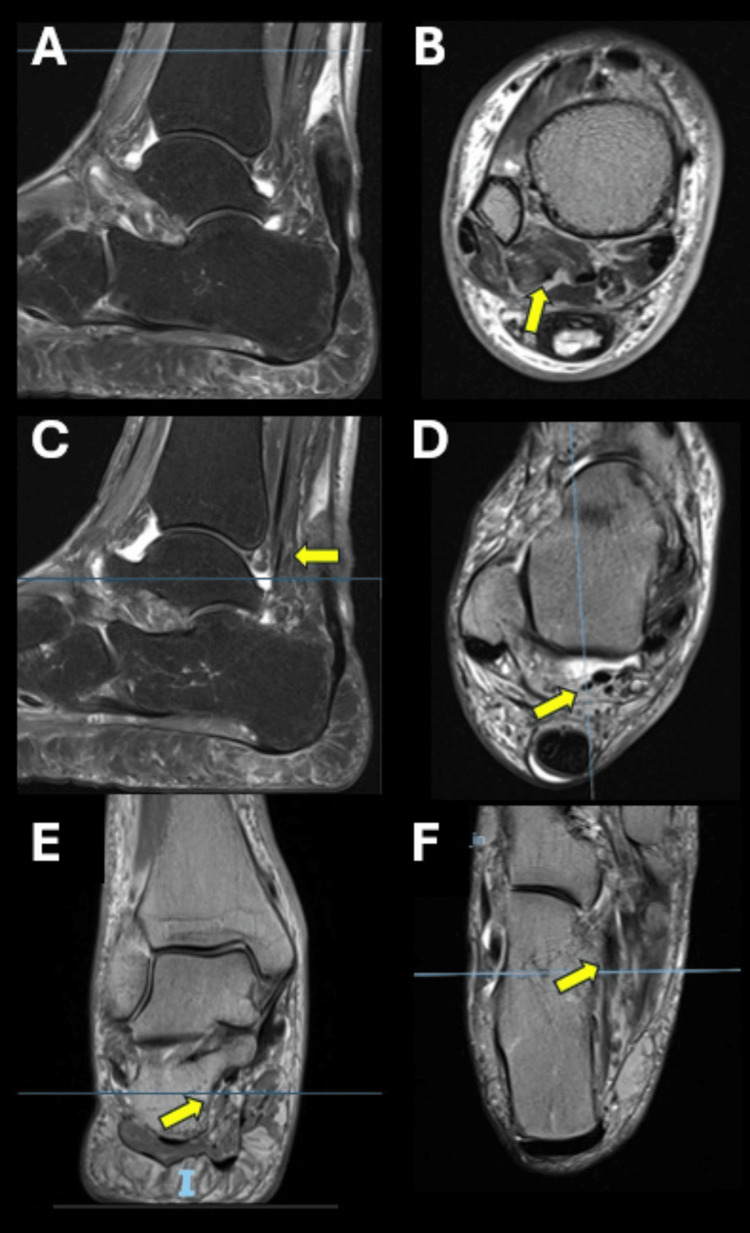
Right ankle MRI: (A-F) cross-sectional study including sagittal, coronal, and axial sequences demonstrating the peroneocalcaneus internus tendon (yellow arrows).
